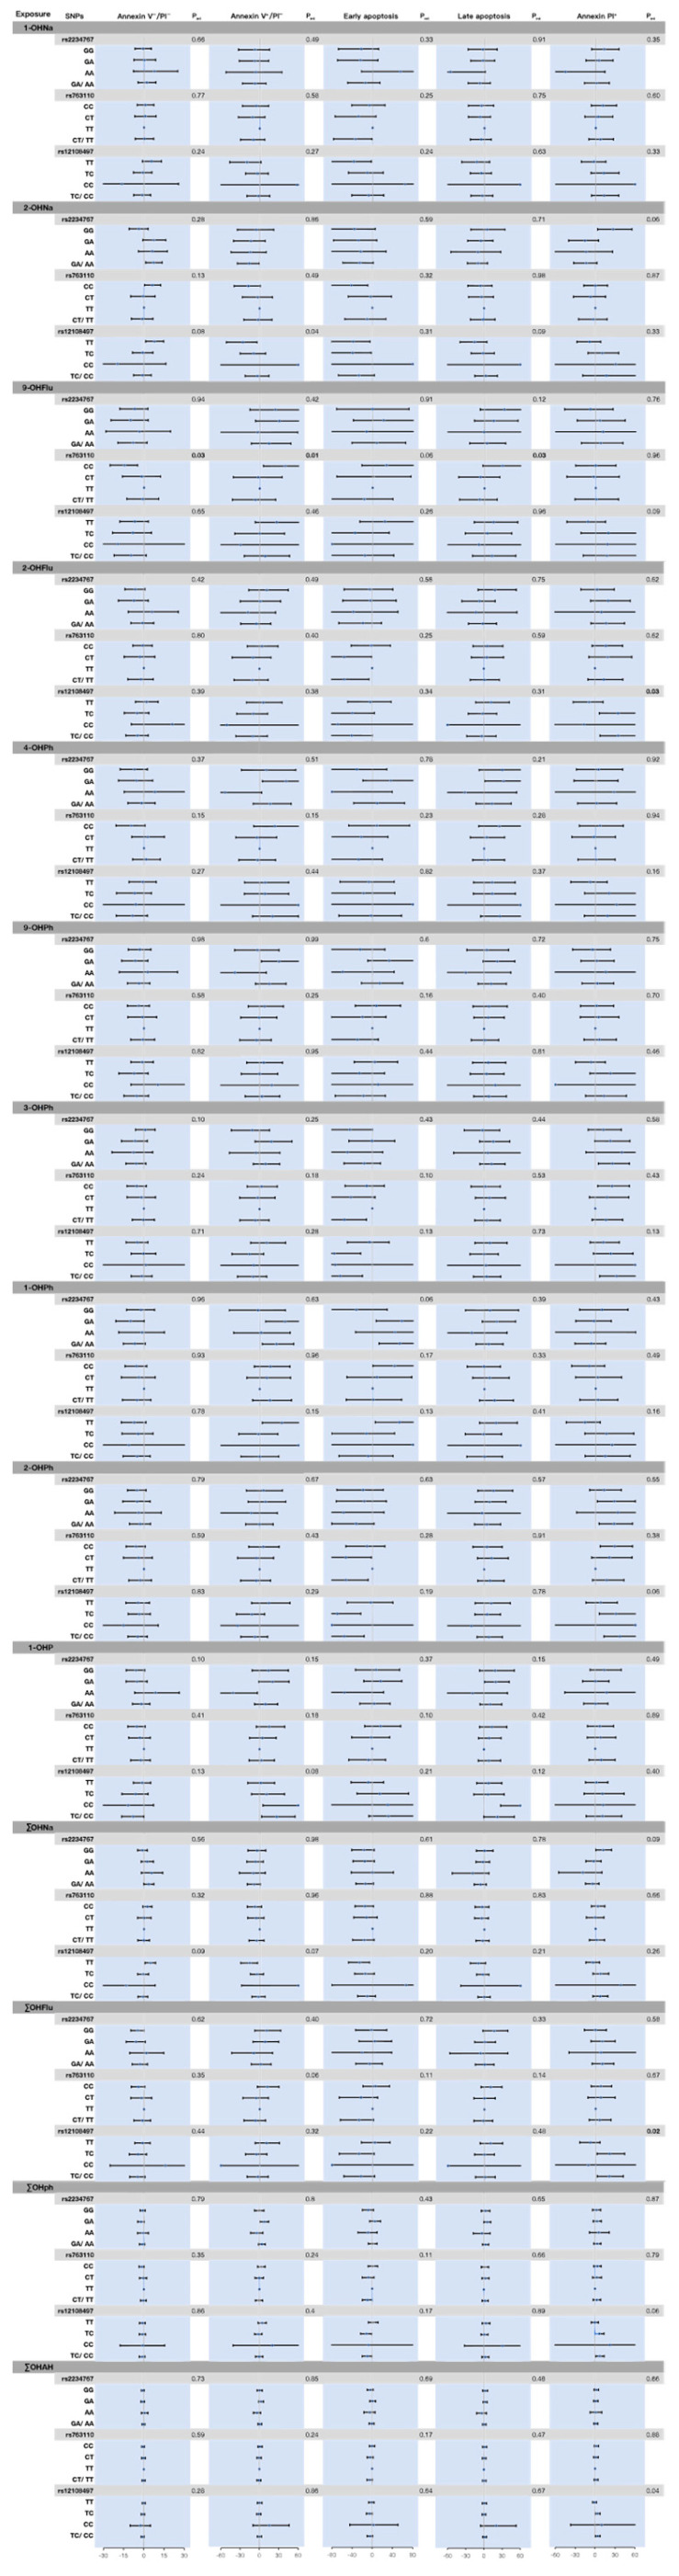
Percentage change in sperm apoptotic parameters associated with PAH exposure across genotypes. Multiple linear regression analyses were used, correcting for age, BMI, pregnacy, income, education, duration of abstinence, smoking status and alcohol consumption. The FasL rs763110 TT variant had insufficient sample numbers and was therefore excluded from analysis. Fas rs2234767 GG, FasL rs763110 CC, and caspase-3 rs12108497 TT are wild-type homozygote; Fas rs2234767 GA, FasL rs763110 CT, and caspase-3 rs12108497 TC are heterozygote; Fas rs2234767 AA, FasL rs763110 TT, and caspase-3 rs12108497 CC are Variant homozygote; Fas rs2234767 GA/AA, FasL rs763110 CT/TT, and caspase-3 rs12108497 TC/CC are combined genotype.
